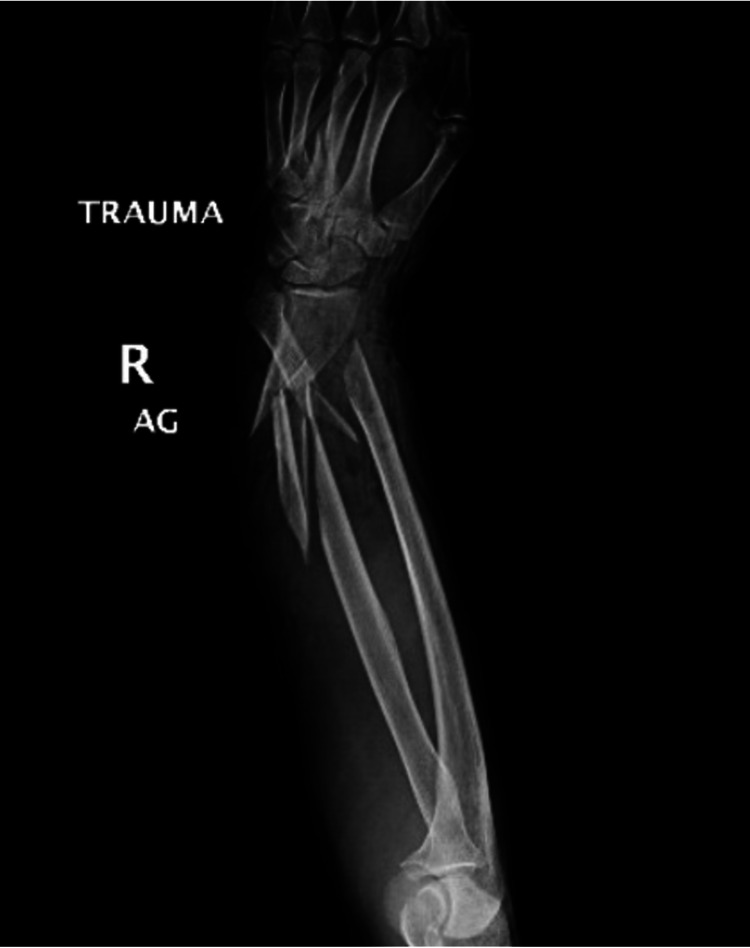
Radiograph of right upper extremity demonstrating ulna, radius, fourth metacarpal, and fifth metacarpal fractures R - Right AG - Silver
